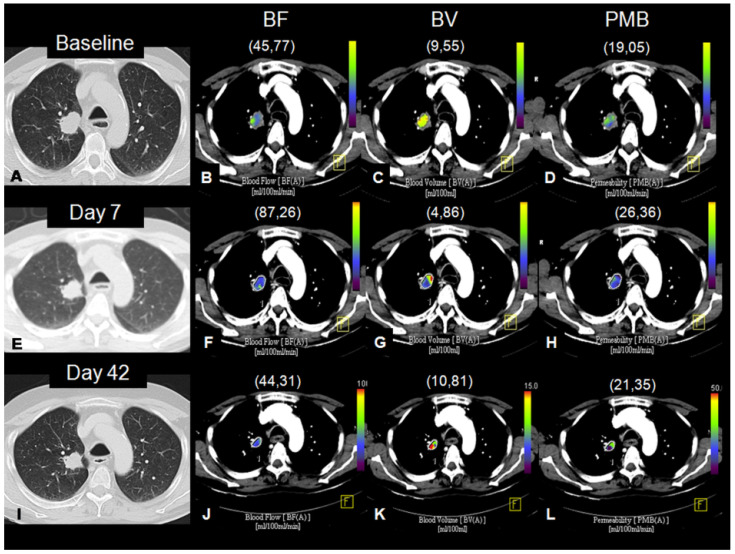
Axial thoracic CT of a 52-year-old man with stage IVa NSCLC showing a lung tumor in the right upper lobe, at baseline (A), day +7 (E), and day +42 (I). Perfusion CT of the lesion with parameters: Blood Flow, BF, (mL/100 mL/min) at baseline (B), day +7 (F), and day +42 (J); Blood Volume, BV, (mL/100 mL) at baseline (C), day +7 (G), and day +42 (K); Permeability, PMB, (mL/100 mL/min) at baseline (D), day +7 (H), and day +42 (L). Color-coded maps and quantification showed an early decrease in BV at day +7 with stable disease according to RECIST at day +42.
